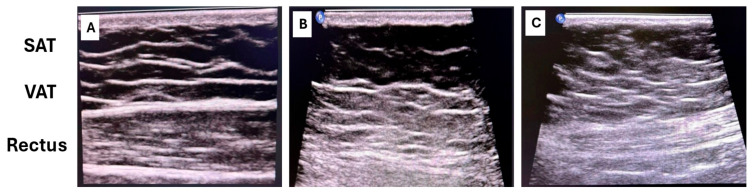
Ultrasound assessment of abdominal subcutaneous fat (SAT), visceral fat (VAT), and rectus muscle morphology across different cardiometabolic profiles. (A) Male, 45 years, no cardiometabolic disease. The ultrasound shows a well-organized layering pattern with clear distinction between SAT and VAT compartments, and a preserved, normally hypoechoic rectus abdominis muscle with appropriate thickness. (B) Male, 39 years, advanced cardiometabolic disease. The image demonstrates poor differentiation between SAT and VAT, with adipose tissue appearing predominantly hyperechoic and structurally disorganized. The rectus abdominis muscle shows marked hypotrophy and sclerosis, appearing diffusely hyperechoic on ultrasound. (C) Female, 70 years, early cardiometabolic impairment. A measurable distinction between SAT and VAT is still present, but the rectus abdominis muscle shows hypotrophy and increased echogenicity consistent with early sclerosis.
